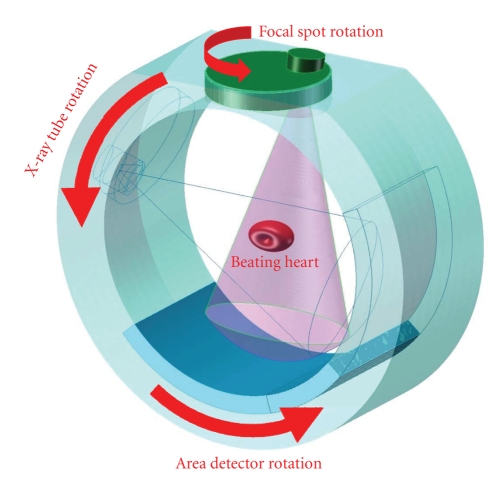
Compositing-circling scanning mode. In such a CT system, the scanning trajectory is a
